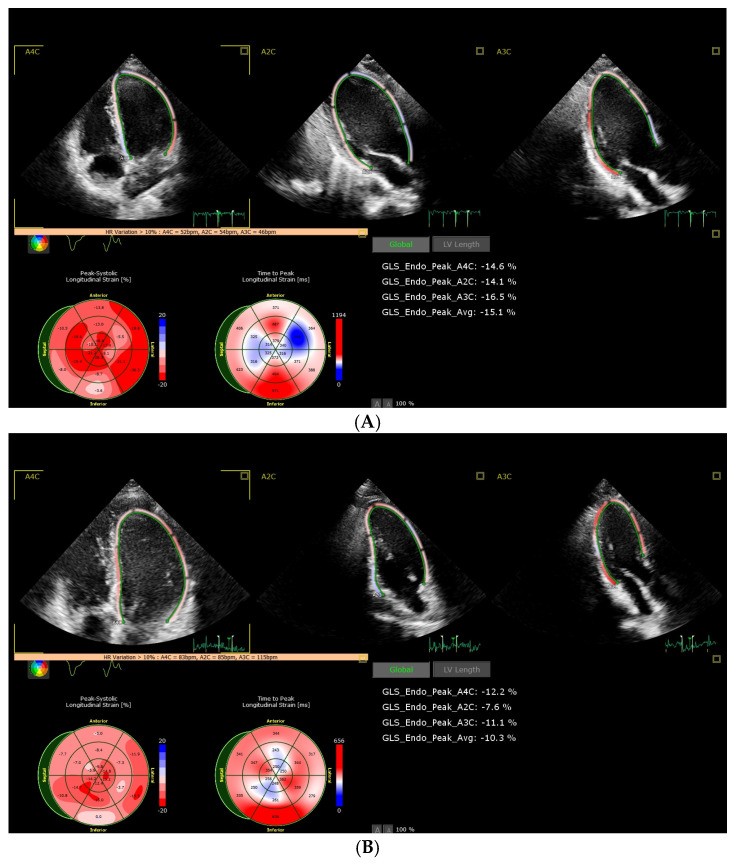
Change in GLS from −15.1% to −10.3%. (A) baseline echocardiography; (B) control echocardiography 12 months after HSCT. GLS: global longitudinal strain, HSCT: hematopoietic stem cell transplantation.
